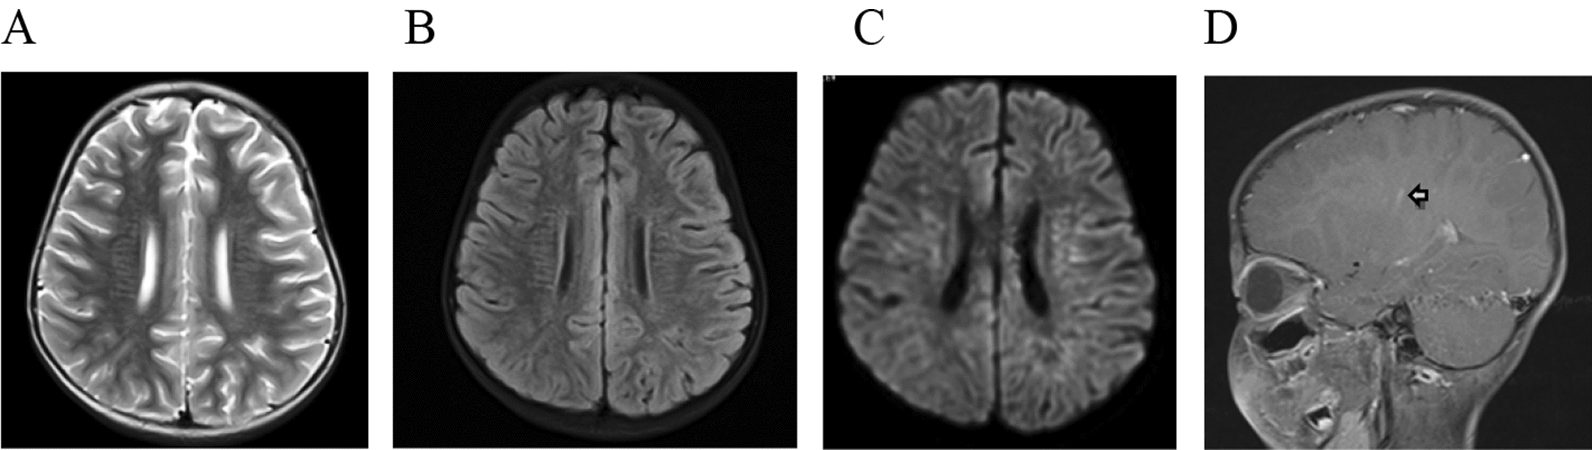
Brain MRI of pediatric patients with autoimmune GFAP astrocytopathy. The abnormal hyperintensity lesions on T2-weighted and FLAIR images were observed in subcortical/around the ventricle white matter (A, B), DWI showed hyperintensity lesions (D). Gadolinium-enhanced brain MRI (T2-FLAIR) showed a linear perivascular radial enhancement pattern (arrow) (D)
